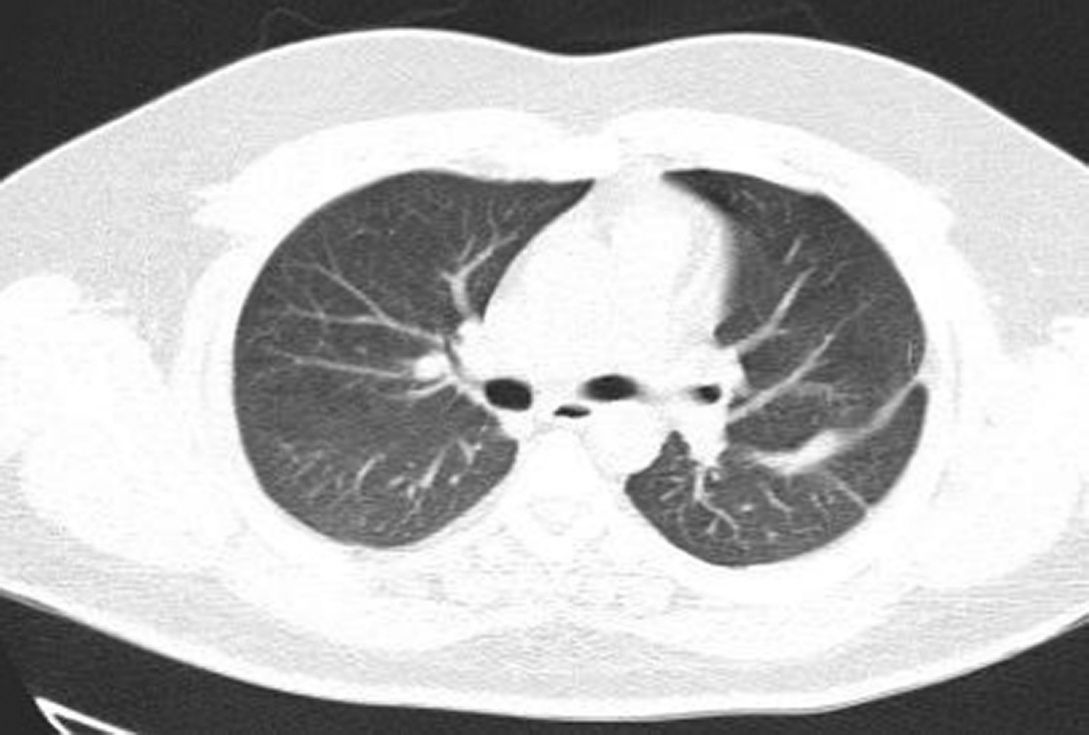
Chest CT scan after complete treatment
